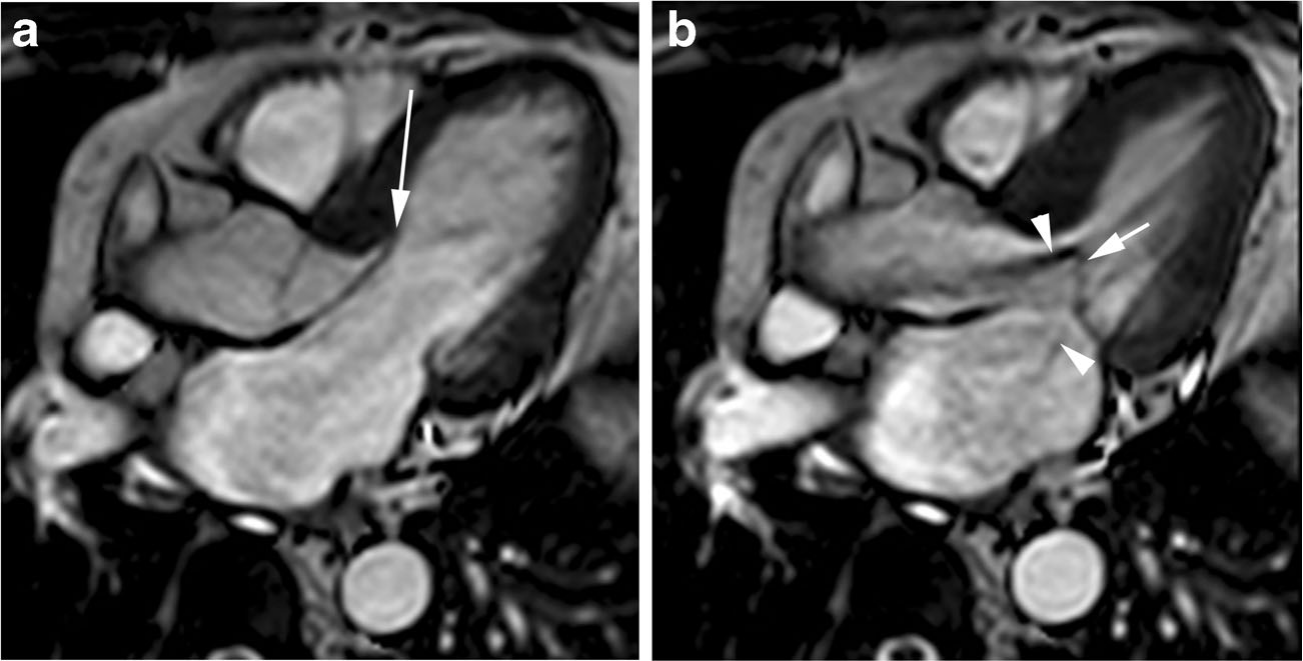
Abnormalities of mitral apparatus in classic HCM phenotype. 44-year-old woman with obstructive HCM, systolic anterior motion of the anterior leaflet of the mitral and mitral regurgitation. Horizontal long-axis SSFP cine MR images at (a) end-diastole and (b) mid-systole show an elongated anterior mitral leaflet (large arrow) with anterior displacement (small arrow) at mid-systole and turbulent-velocity jets (arrowheads) within the LV outflow tract and into the left atrium due to LV outflow tract obstruction and mitral regurgitation, respectively
